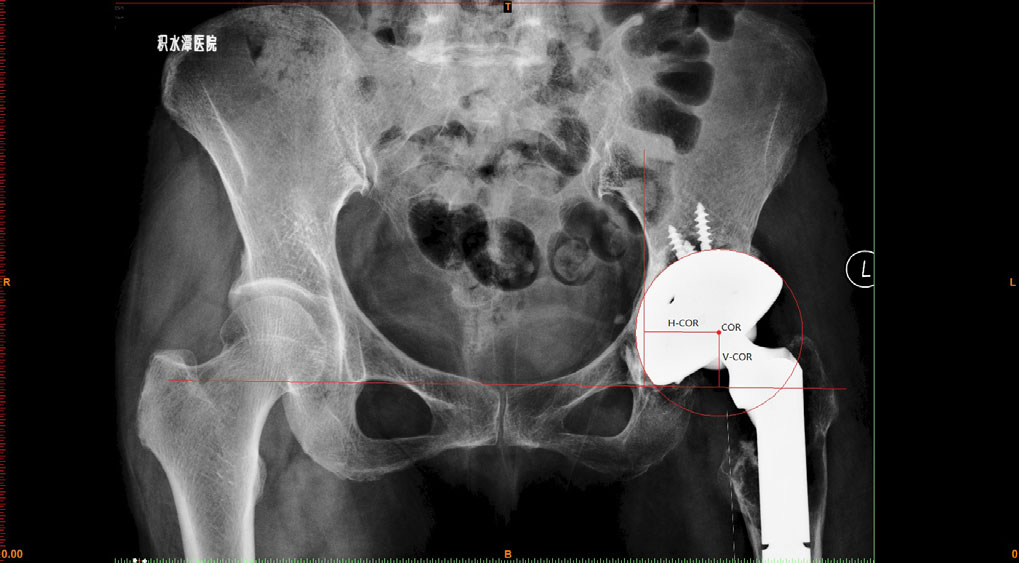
Radiographic measurements using Mimics 10.0 software on an anteroposterior (AP) pelvic radiograph. COR, center of rotation; H‐COR, horizontal distance of the COR; V‐COR, vertical distance of the COR.
